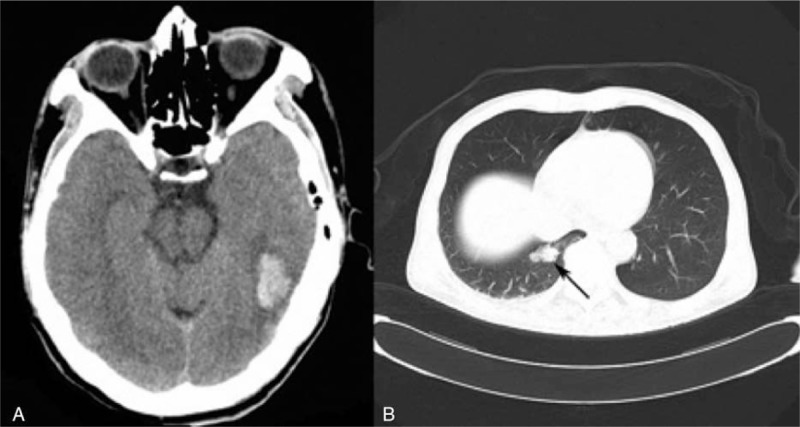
Brain CT and lung CT. Brain CT (A) showed hemorrhage in the left temporal occipital lobe. Lung CT (B) showed a high density lesion in the right posterior lobe. CT = computed tomography.
